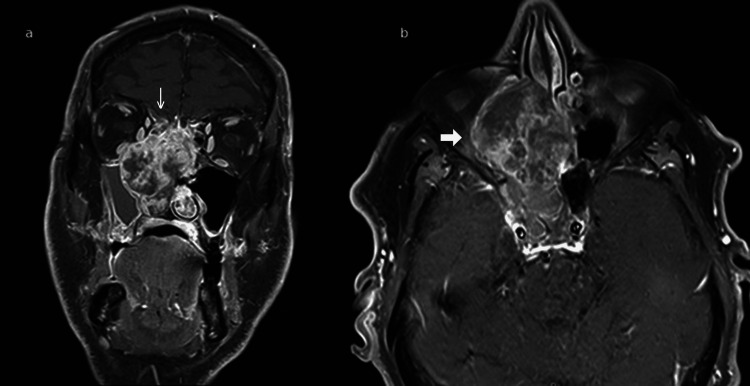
Post-contrast T1-weighted with fat saturation MRI findings (a) coronal view showing an infiltrative mass extending into the left orbit and skull base (thin arrow); (b) axial view demonstrating the same lesion invading adjacent bony and soft tissue structures (thick arrow).
